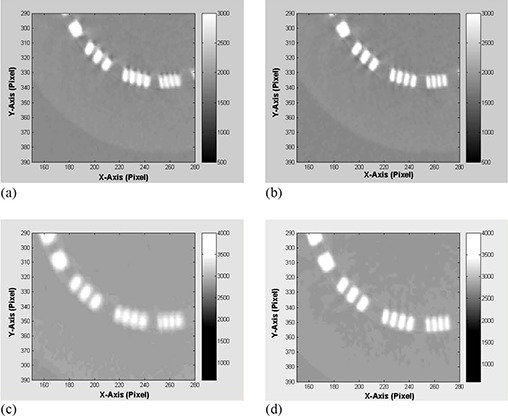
(a) and (b) show comparison between CBCT position resolution slices reconstructed from projections before and after OBI sag correction for HF scan; (c) and (d) are comparisons of position resolution for FF scans.
